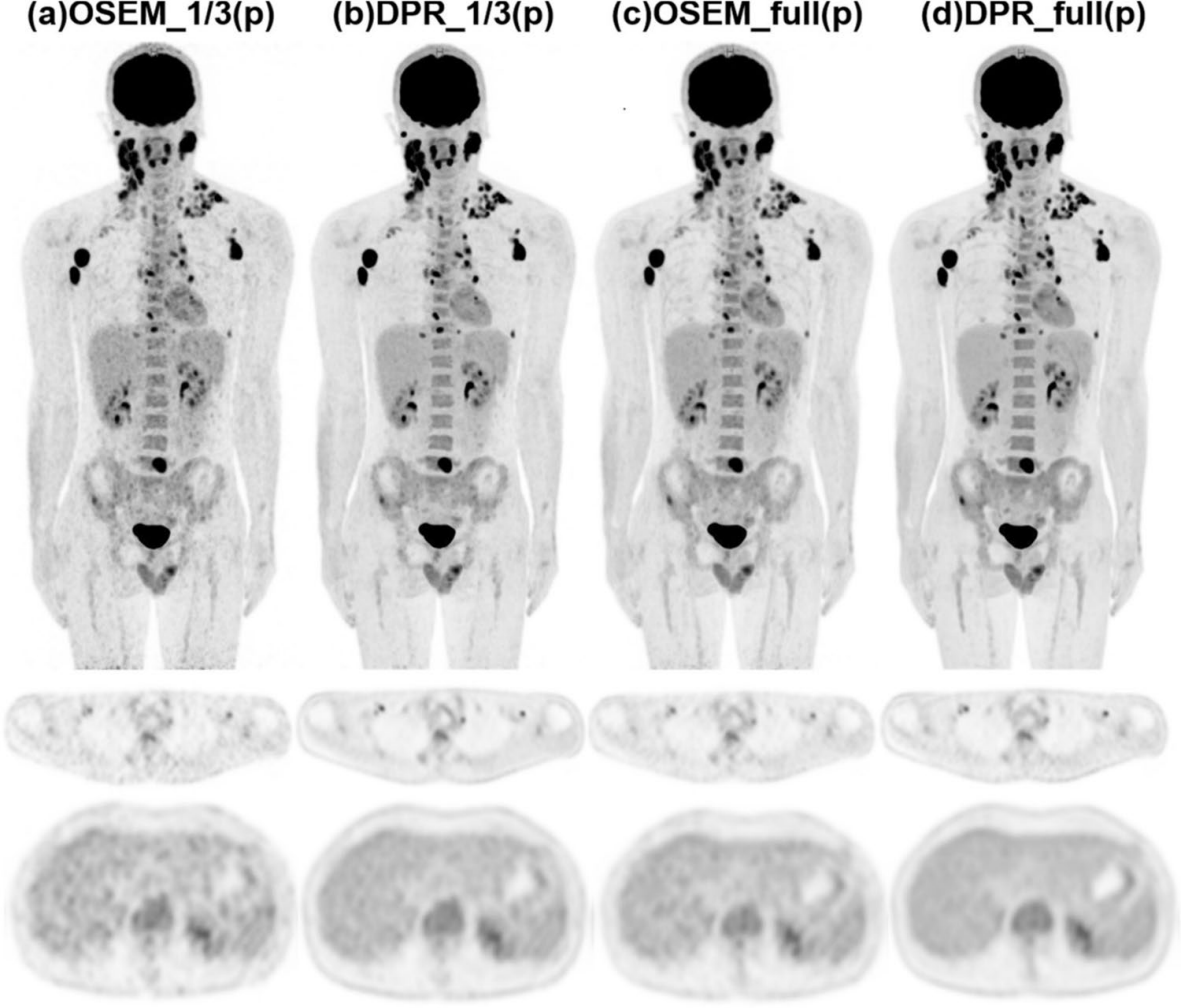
Images of 31-year-old male patient diagnosed with Hodgkin’s lymphoma, reconstructed using HYPER DPR and OSEM. We reconstructed PET data using both algorithms at full and one-third acquisition durations to enable a comprehensive comparison. Image noise is reduced and edge definition is enhanced in ^18^F-FDG PET images reconstructed by HYPER DPR compared with OSEM at the same acquisition time. (Reprinted with modification from Wang et al. [126]. Copyright © 2022, Wang et al. licensed under CC BY)
